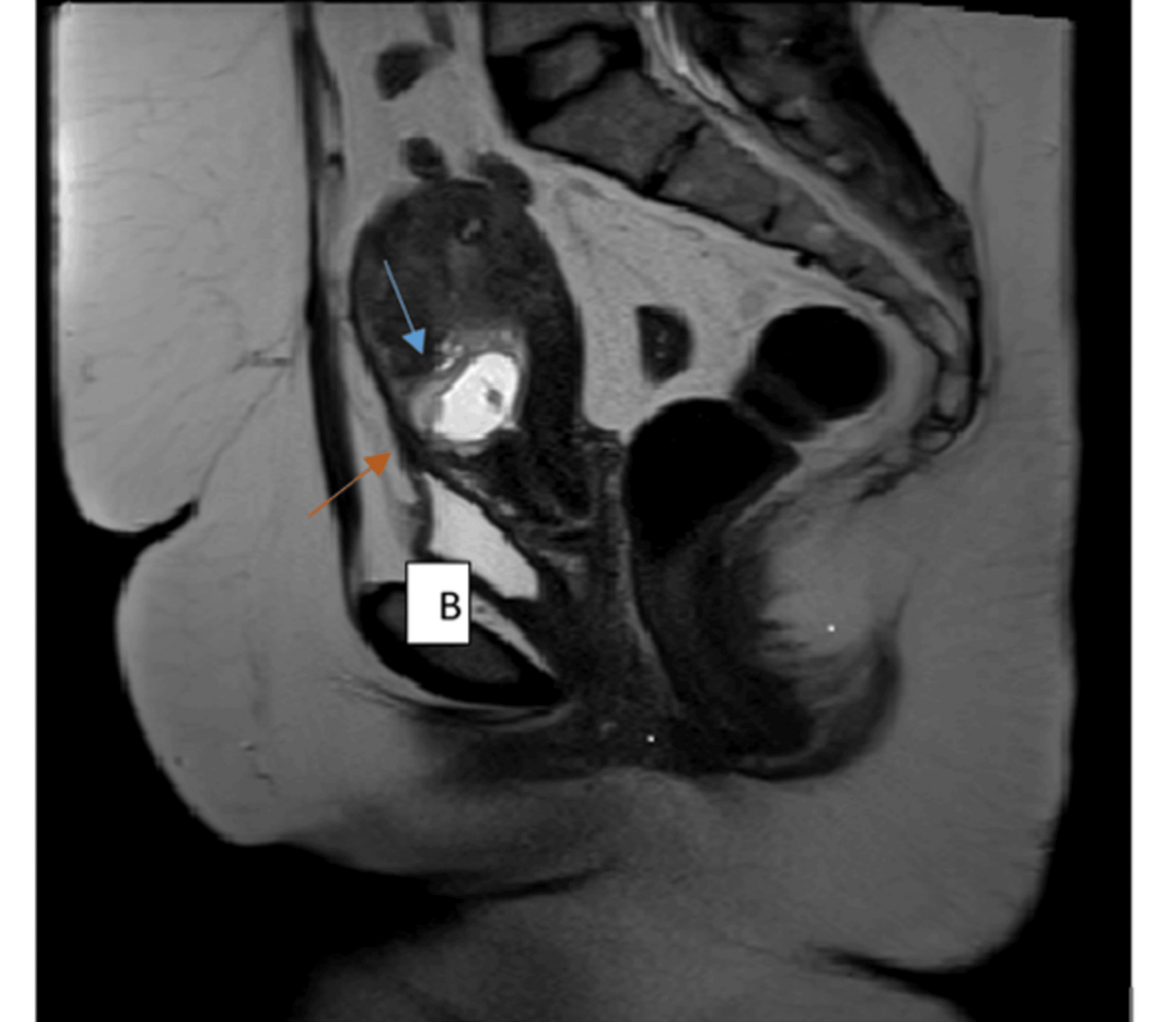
Coronal T2-weighted and axial haste T2W MRI of the pelvis for the same patient showing a partial septated uterus A sagittal T2-weighted MRI of the pelvis demonstrates a gestational sac (blue arrow) implanted within the anterior wall of the lower uterine segment in the region of the previous cesarean scar. Bladder wall integrity is preserved (B).
